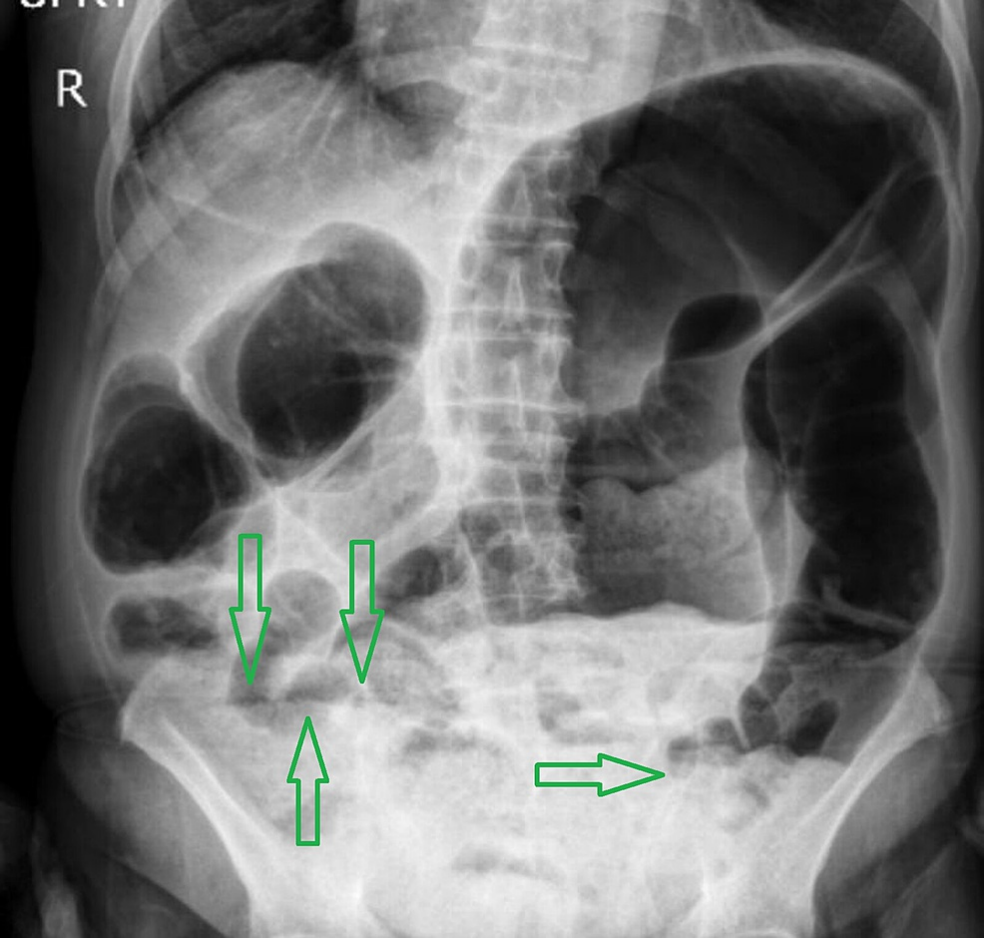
Upright abdominal X-ray Green arrows show air-fluid levels
